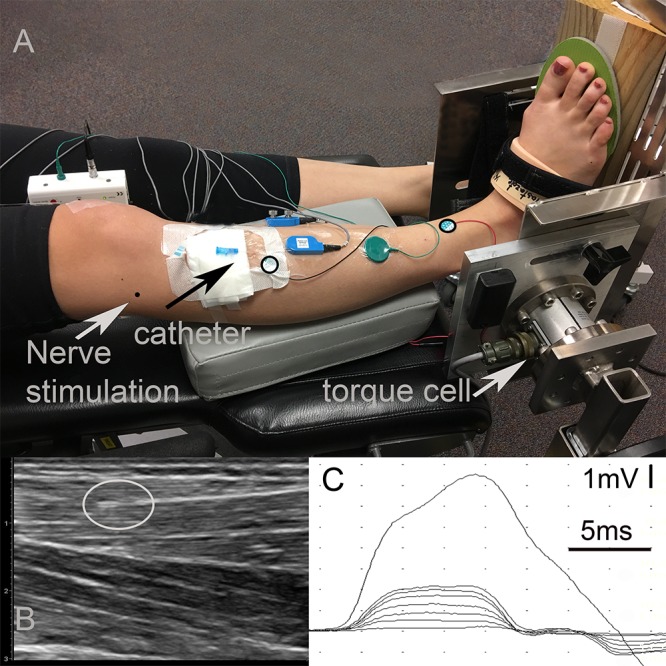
Experimental data collection. (A) Setup: The right foot of the participant strapped into a custom torque measurement system. The center of the torque cell was aligned with the ankle axis using a laser pointer whose light was in line with the lateral malleolus. A wooden wedge with an angle of 20° was placed underneath the foot in order to promote the optimal line of action of the tibialis anterior (TA) muscle. Black circles on the TA show the locations of the anode and cathode of the CMAP surface recording electrodes. The black arrow shows the entry of the catheter. The tip of the sensor is below the black circled electrode inside the muscle. The gray arrow shows the entry of the fine-wire needed for fibular nerve stimulation. Note that data collected from surface EMG electrodes and fine-wire EMG electrodes shown in the picture were not included into this study. (B) Ultrasound image of the TA identifying the location of the intramuscular pressure (IMP). The gray circle shows the tip of the IMP sensor. (C) Time dependent tracing of the compound muscle action potential (CMAP) for different levels of fibular nerve stimulation.
